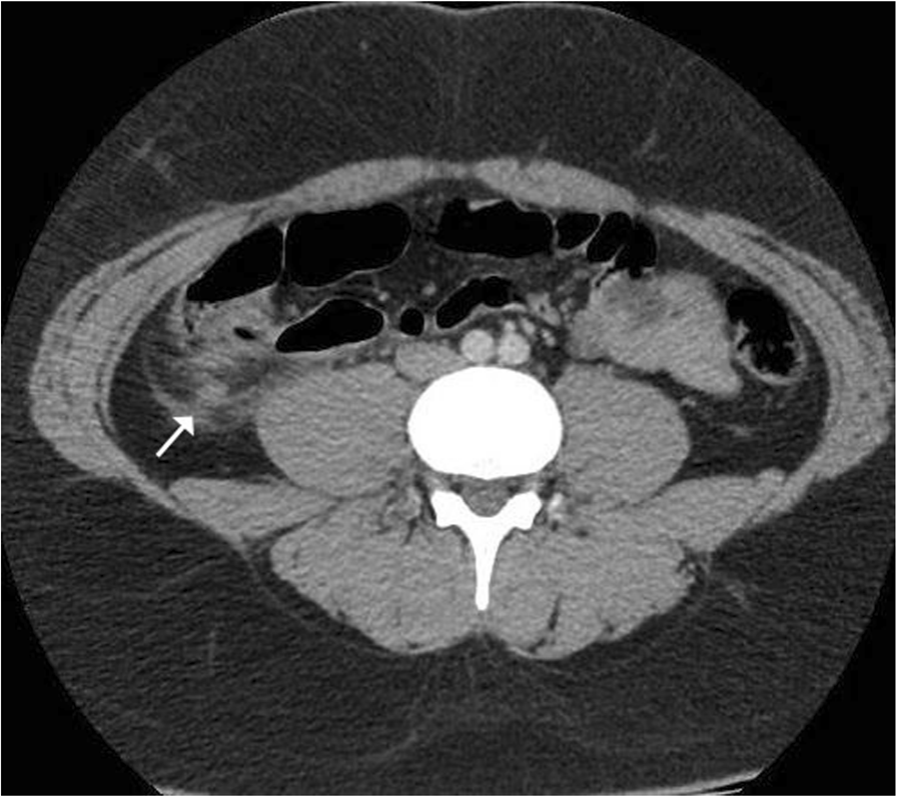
Abdominal CT scan. In the right lower quadrant, inflammation in the pericecal region and tip of the remnant appendix tissue (arrow) is observed
